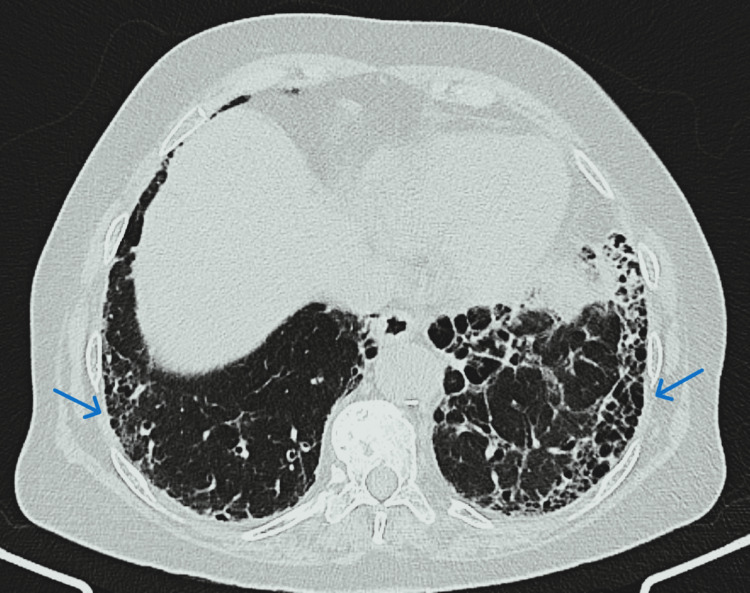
High-resolution computed tomography (HRCT) thorax revealed multiple areas of honeycombing involving bilateral lower lobes (left > right), and basal subpleural areas of fibrosis (likely suggestive of interstitial lung disease) consistent with definite UIP pattern.
